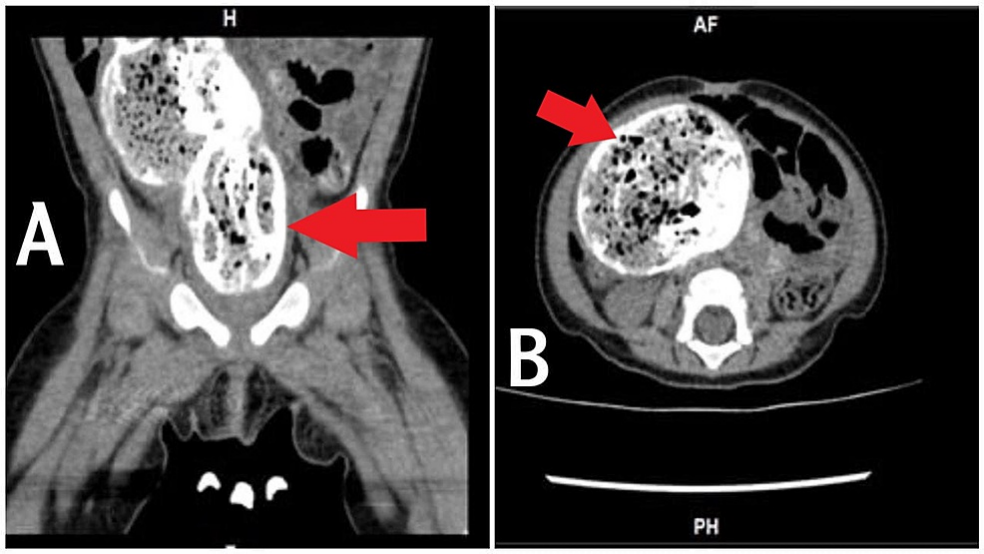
CT of the pelvis without contrast showed megacolon with a high position of the rectal pouch above the level of the puborectalis muscle at the level of S2/S3 A puborectalis muscle cyst is seen with thickened walls measuring around 1×0.5 cm. There is an S5 hemivertebra. The anterior position of the anal canal had irregular, thickened levator ani muscles. There is compression on the bladder by the megacolon. Fecal matter and residual barium contrast are seen.
